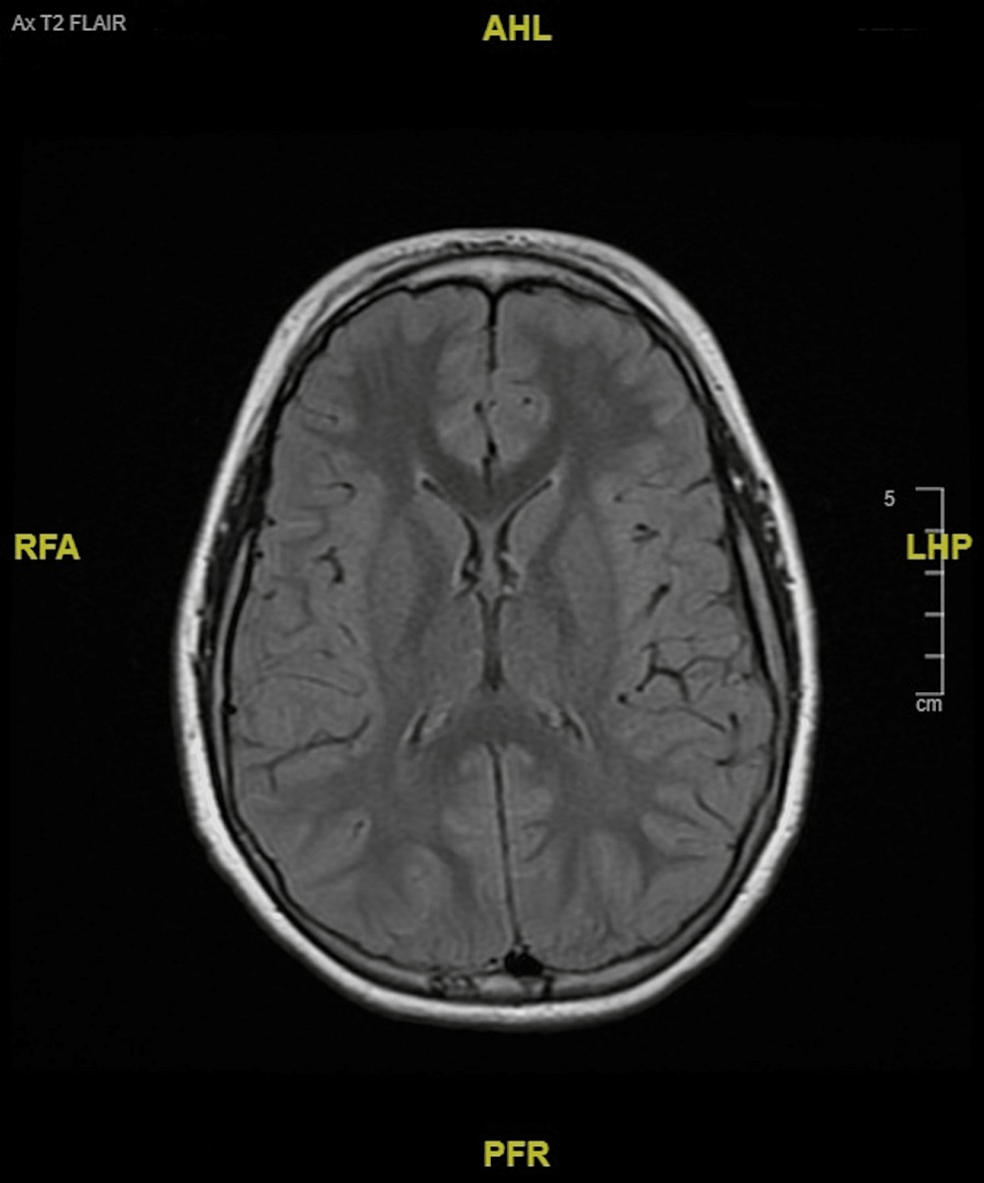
MRI brain shows normal ventricles The image shows a T2-weighted FLAIR sequence taken on a 1.5-Tesla scanner. Dotarem intravenous contrast (12 mL) was administered. MRI brain with contrast found no acute pathology. FLAIR: Fluid-attenuated inversion recovery.
